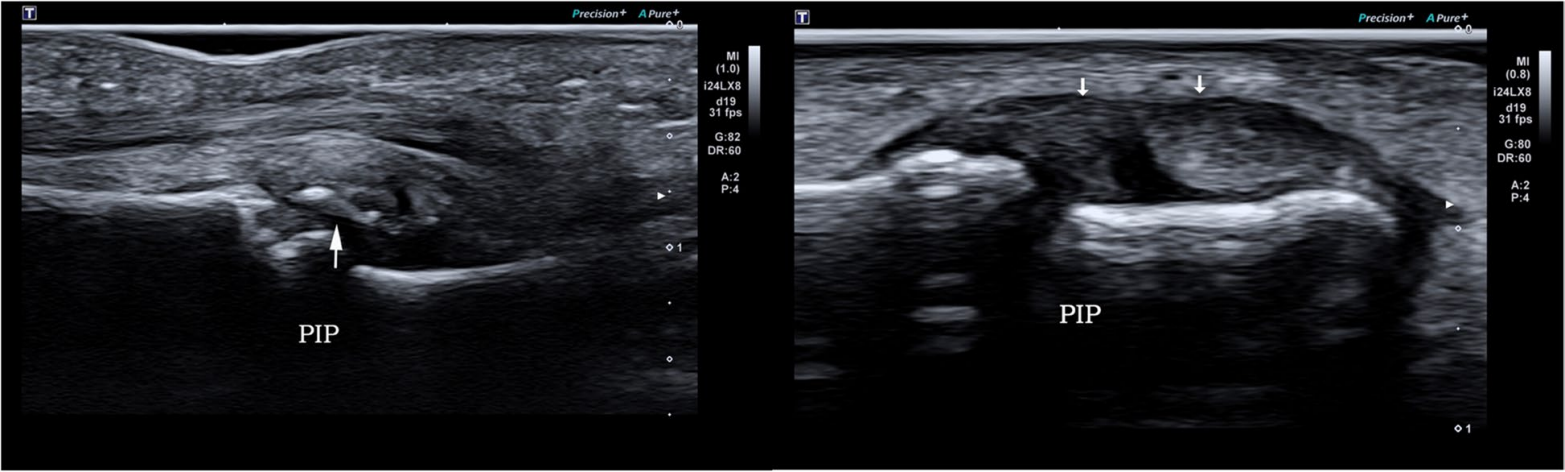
Volar plate injury accompanied by lateral collateral ligament injury. High frequency ultrasonography image of type A volar plate injury of a 33-year-old male accompanied by lateral collateral ligament injury. The arrow in the left figure indicates the avulsion bony fragment of the middle phalangeal base, and the arrow in the right figure indicates the disruption of the lateral collateral ligament. PIP: proximal interphalangeal joint
